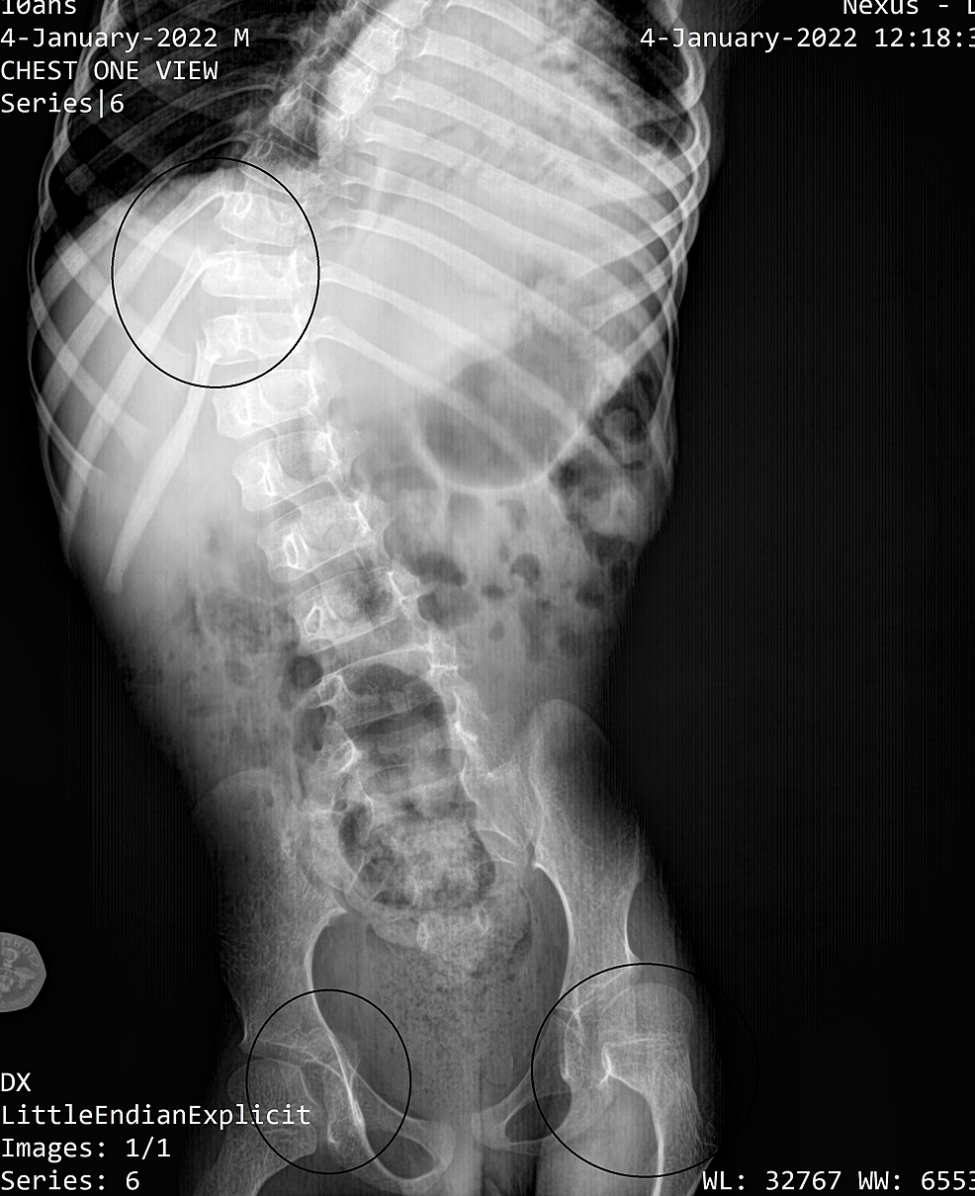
Vertebral column and pelvis (x-ray findings)
